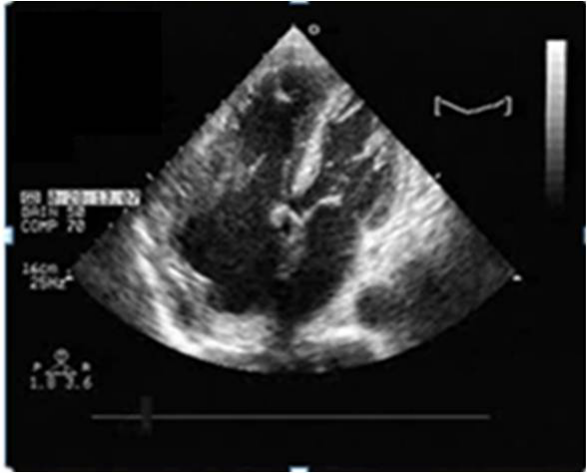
A transthoracic echocardiography four chamber view showing a reduction of the size of the mass.
